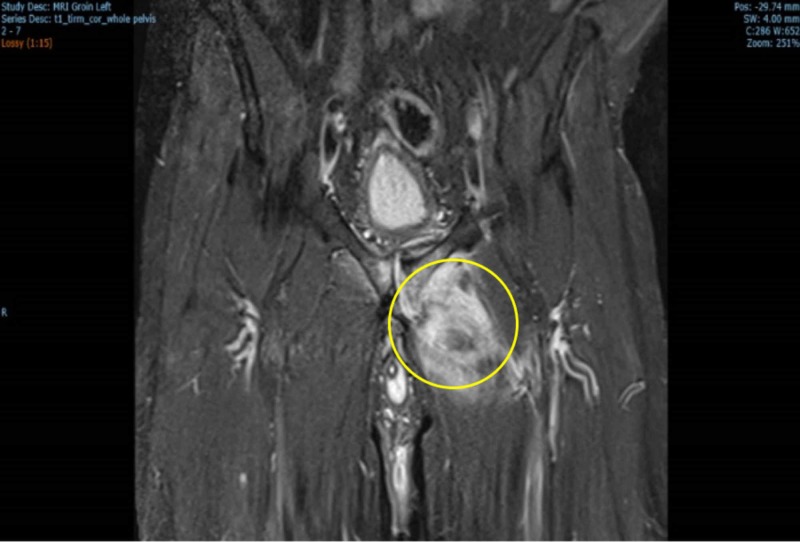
Coronal MRI image shows that the previously visualized fluid collection within the left adductor muscle compartment has partially resolved two months post-catherization. There remains however significant oedema within the muscle fibres. The ongoing communication from the bladder neck into the pubic symphysis is evident.
